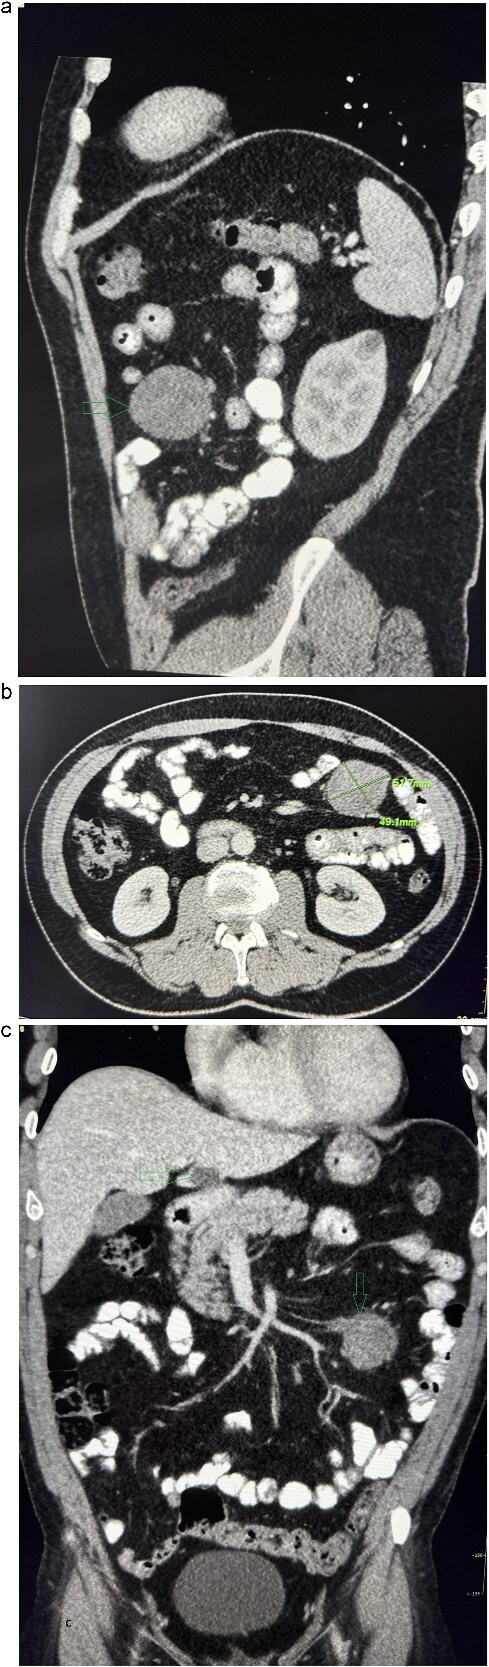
Contrast-enhanced abdominal CT. (a) The sagittal section presents a tumor mass at the root of the mesentery in the left mesogastrium. (b) The axial section presents a tumor mass measuring 52×49 mm in diameter. (c) The coronal section presents a tumor mass at the root of the mesentery in the left mesogastrium and suspected metastatic liver lesion.
